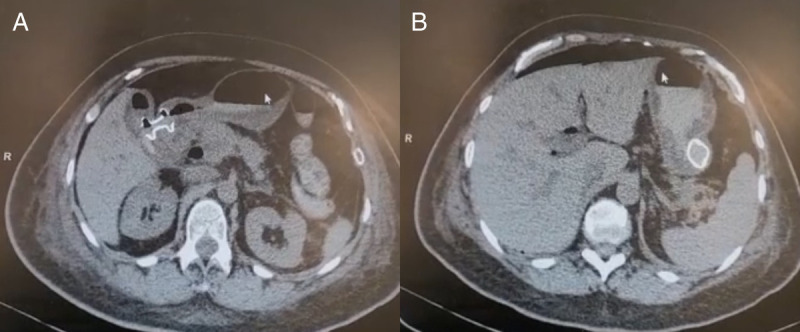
Postprocedural CT scan. A, Tomographic imaging EUS-guided cholecystogastrostomy with LAMS in adequate position. B, Tomographic imaging of EUS-guided gastroenterostomy with LAMS in adequate position. CT: Computed tomography; LAMS: Lumen-apposing metal stent.
